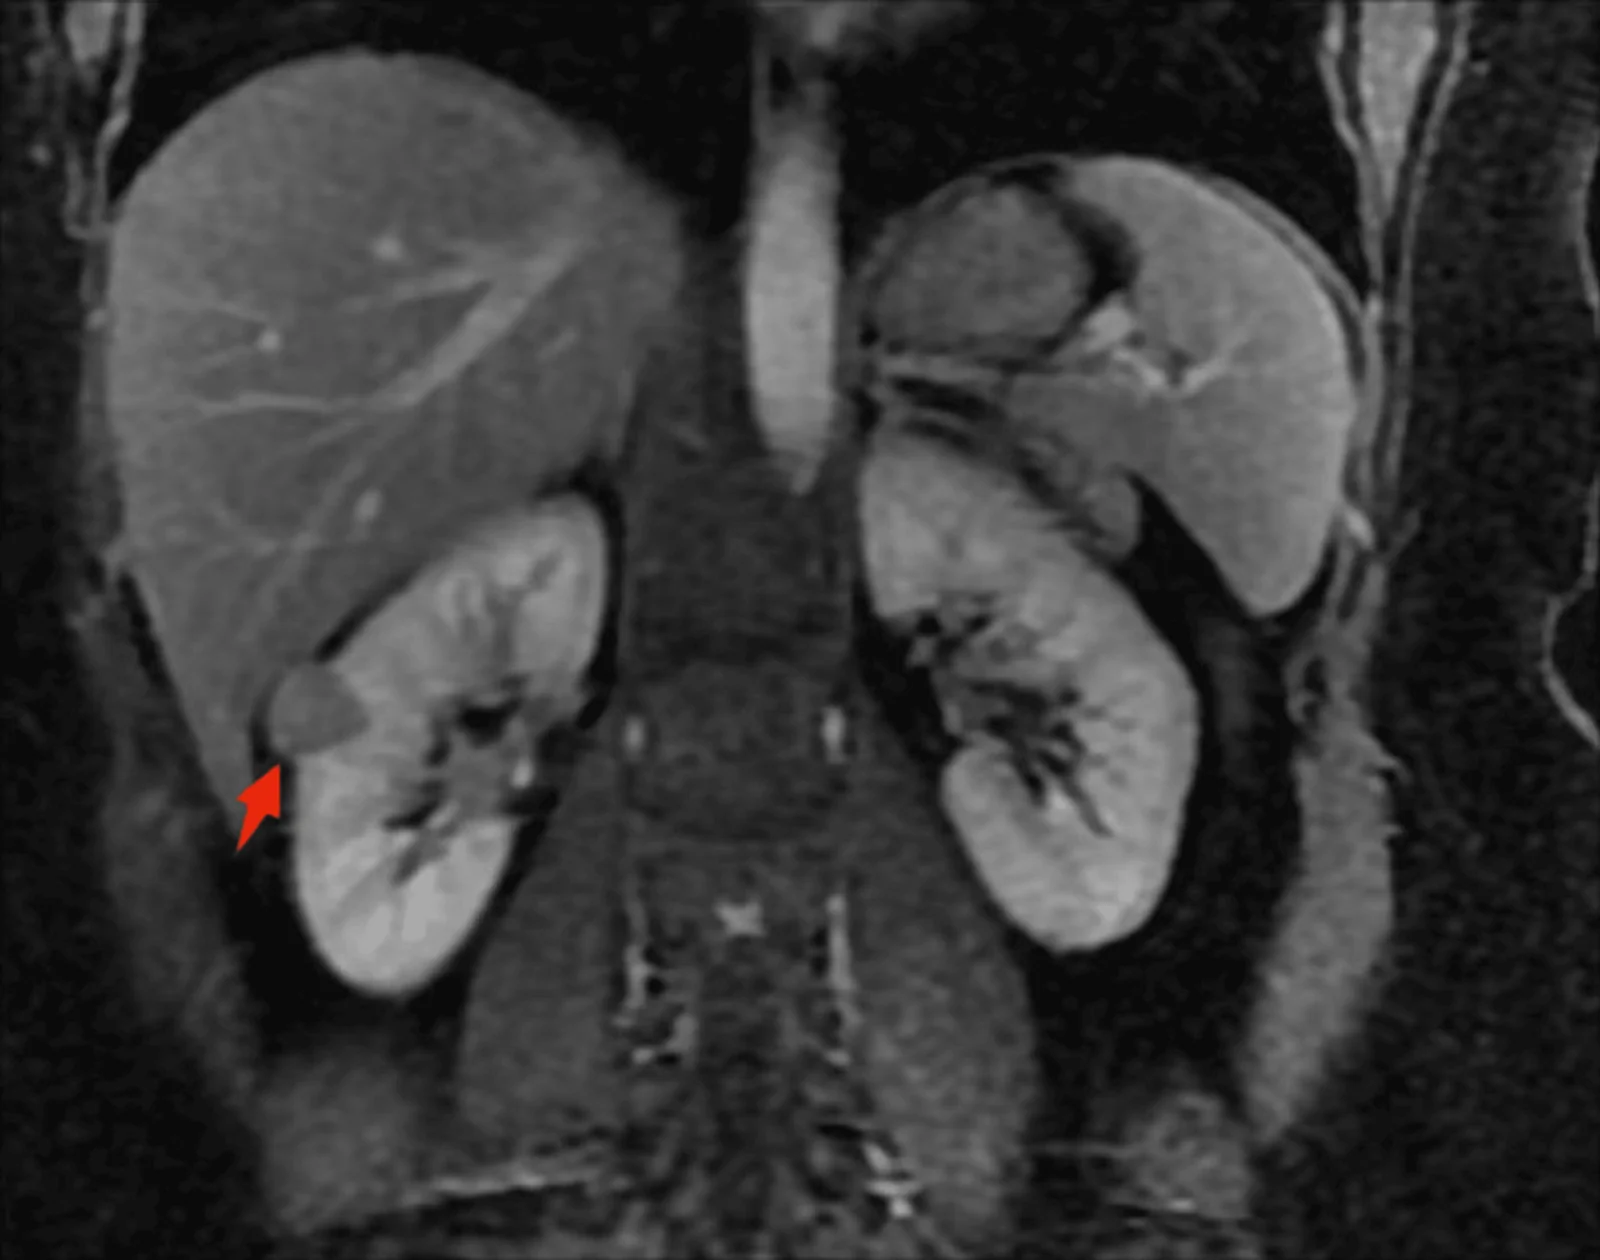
Delayed phase of dynamic contrast-enhanced MRI The mass appears as a relatively low-signal-intensity lesion because the contrast agent is cleared from it, as indicated by the arrow MRI: magnetic resonance imaging
